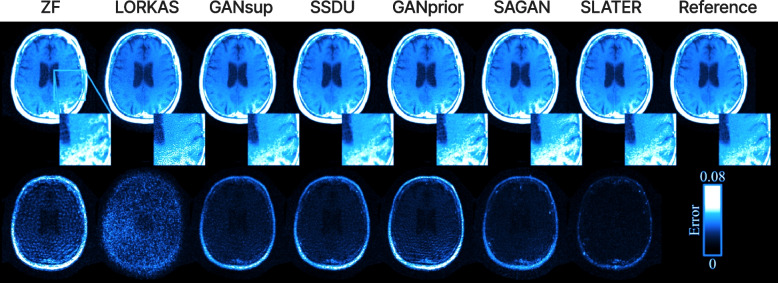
Top: Different reconstruction methods from *T*_1_ weighted acquisition of the fast MRI using different methods. ZF is a traditional Fourier method [70]. LORKAS [71, 72], GAN_*sub*_ [73], SSDU [74], GAN_*prior*_ [75], and SAGAN [76] are generative adversarial network (GAN) reconstruction-based methods. SLATER is a ViT-based method [69]. Bottom: Reconstruction error map [69]
